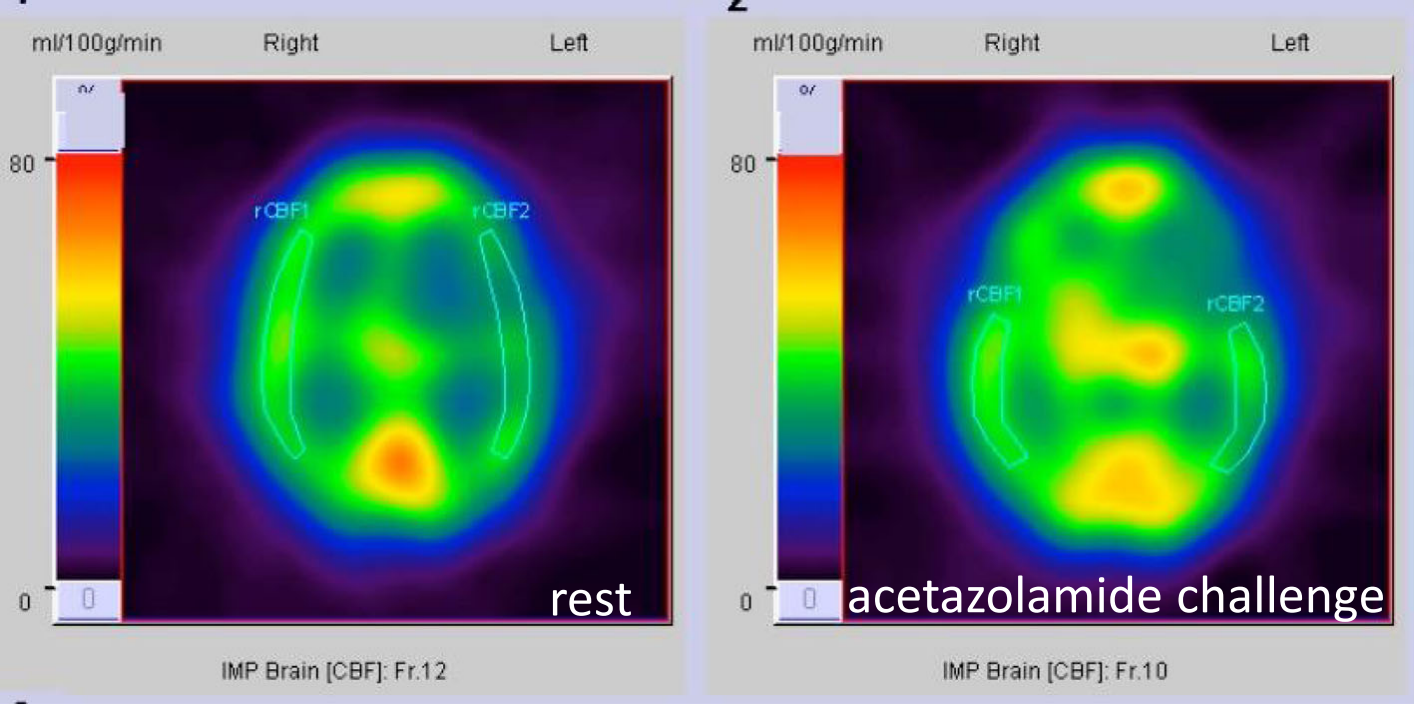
Brain Perfusion SPECT (123I-IMP). Preoperative SPECT (123I-IMP) revealed significant reduction in cerebral blood flow at rest and reactivity to acetazolamide, particularly in the left MCA territory (rest and acetazolamide). SPECT: single-photon emission computed tomography. 123I-IMP: N-isopropyl-p-[123I]iodoamphetamine
